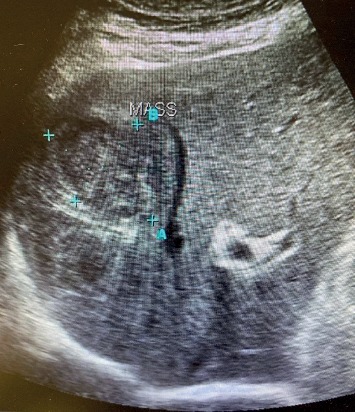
Ultrasound liver showing the heterogeneous mass suggestive of abscess.
